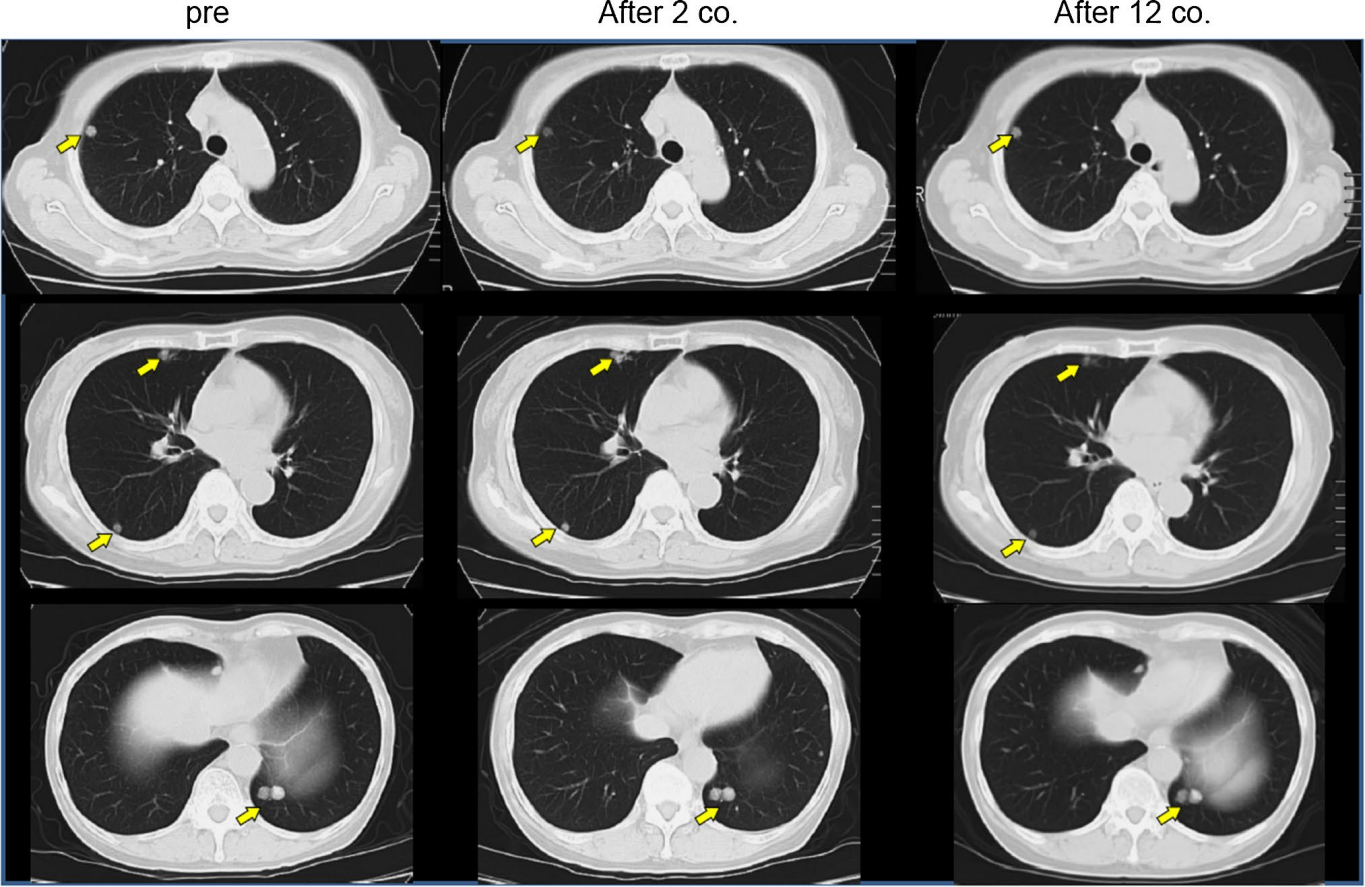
Chest CT image of a long SD case. Chest CT image of case 1, showing multiple lung metastases as indicated by* arrows* before the vaccine treatment. After 2 and 12 courses of peptide vaccine (0.5 mg/body) treatment, the sizes of multiple lung metastases were unchanged
